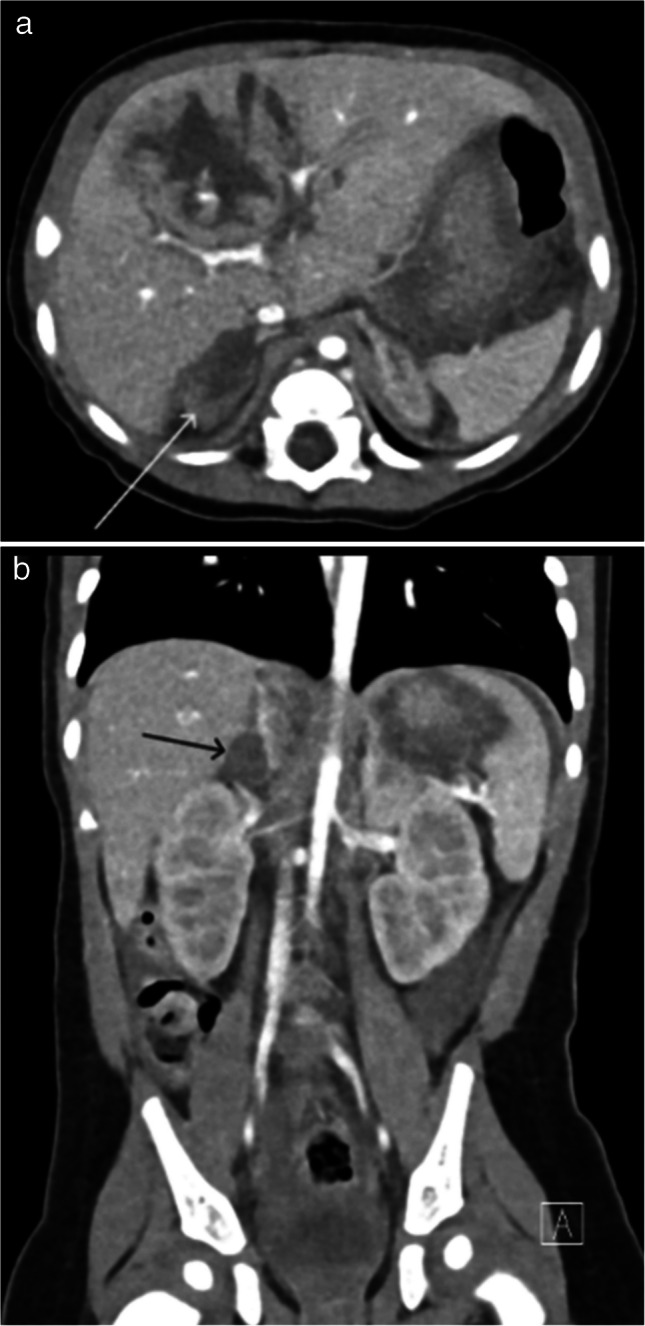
A 5-month-old male who was brought to the emergency department by his aunt for sleepiness and bruising over face, neck, chest, abdomen, and thighs. Further workup revealed bilateral rib fractures of different ages and elevated liver enzymes. a Axial contrast-enhanced CT scan of the abdomen in portal venous phase shows an oval, hypodense right adrenal hematoma (*white arrow*). The liver shows an area of branching lacerations, consistent with co-existing hepatic injury. b Coronal contrast-enhanced CT image of the same study shows the oval hypodense right adrenal hematoma (*black arrow*) which splays the adrenal limbs
